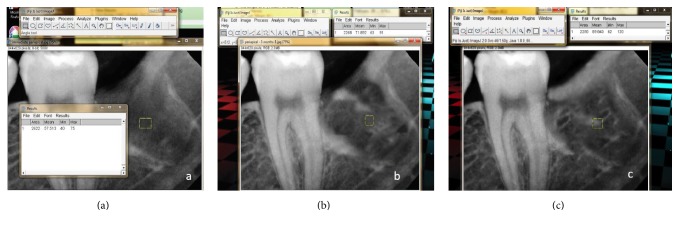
(a) Immediate, (b) 3-month, and (c) 6-month standardized periapical X-ray measuring bone density using ImageJ software (test site).
